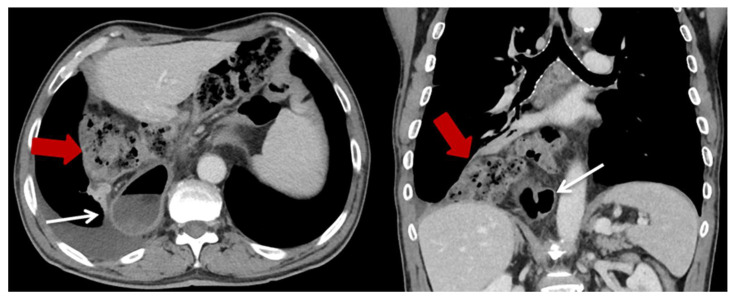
Exemplary CT-scan of a hiatal hernia on the right thoracic side after HMIE. Gastric conduit marked with thin white arrow; herniated colon marked with thick red arrow.
